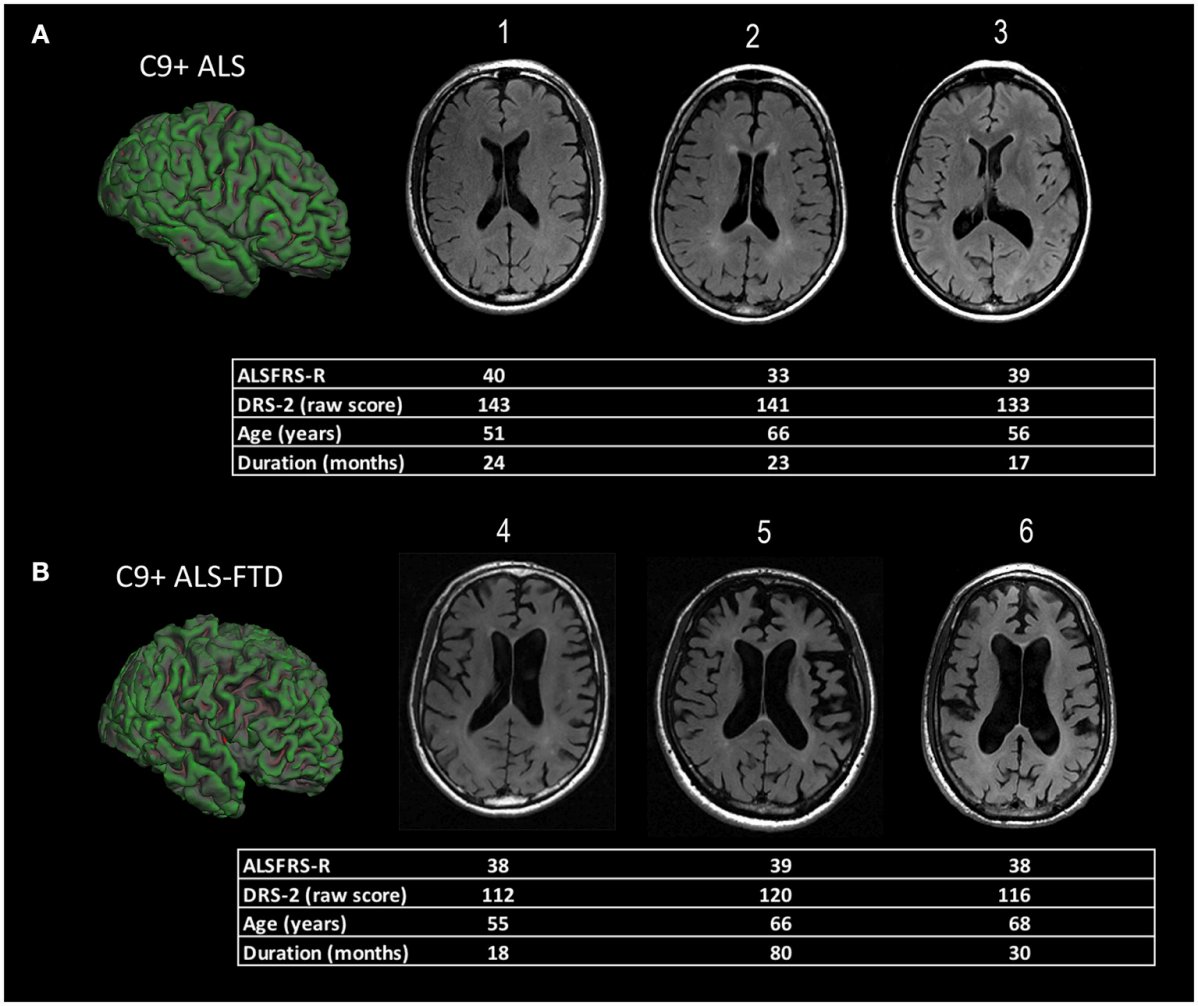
Representative examples of diffuse cortical atrophy in MRI scans of ALS patients with *C9orf72* mutations. The demographic information and scores on motor and cognitive scales are listed below each patient's scan. (A) Compared to age-matched controls, mild ventricular enlargement was seen in C9+ patients 1, 2, and 3 who had ALS, but good cognitive function, as evidenced by their scores on the Mattis Dementia Rating Scale−2 (DRS-2). The surface rendering of one patient [left side of panel (A)] shows sulci in frontal lobe sulci are also mildly enlarged compared to the occipital lobe. (B) C9+ patients 4, 5, and 6 had ALS-FTD with a similar degree of motor dysfunction to those in panel (A), as measured by their ALS functional rating scale revised (ALSFRS-R) scores, but marked cognitive impairment with low DRS-2 scores. There is marked enlargement of ventricles evident in axial slices, as well as enlargement of frontal and temporal sulci in the surface rendering at left of panel (B).
